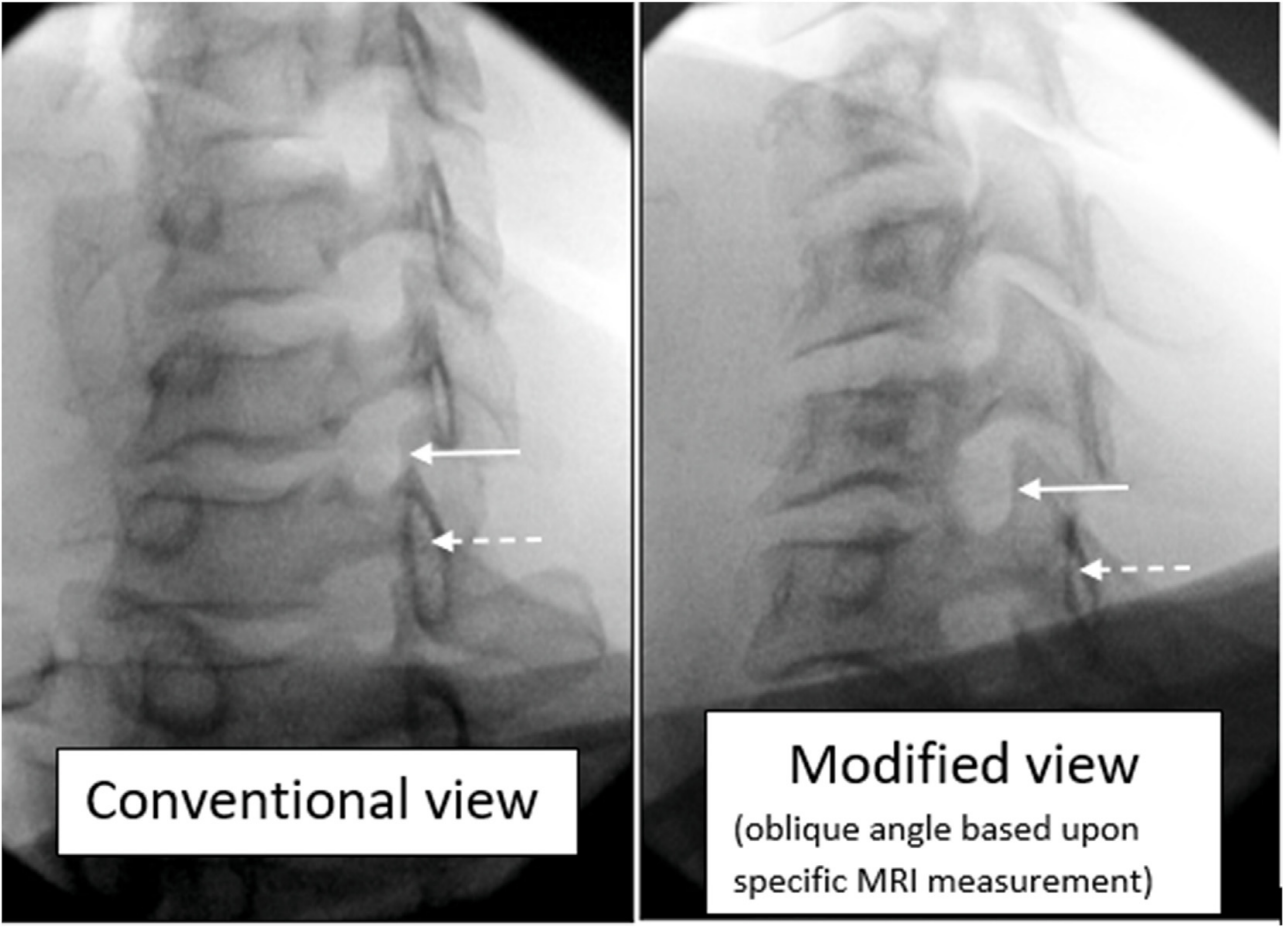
Fluoroscopic oblique images: Conventional view based upon co-alignment of the superior articular process (SAP) (solid arrow) and lamina (dashed arrow). Modified oblique view is based upon the obliquity of the specific angle measurement of the ventral surface of the SAP on MRI.
